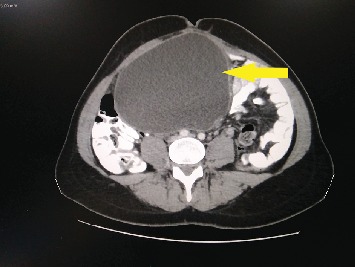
CT scan of the mesenteric cyst (arrow).
